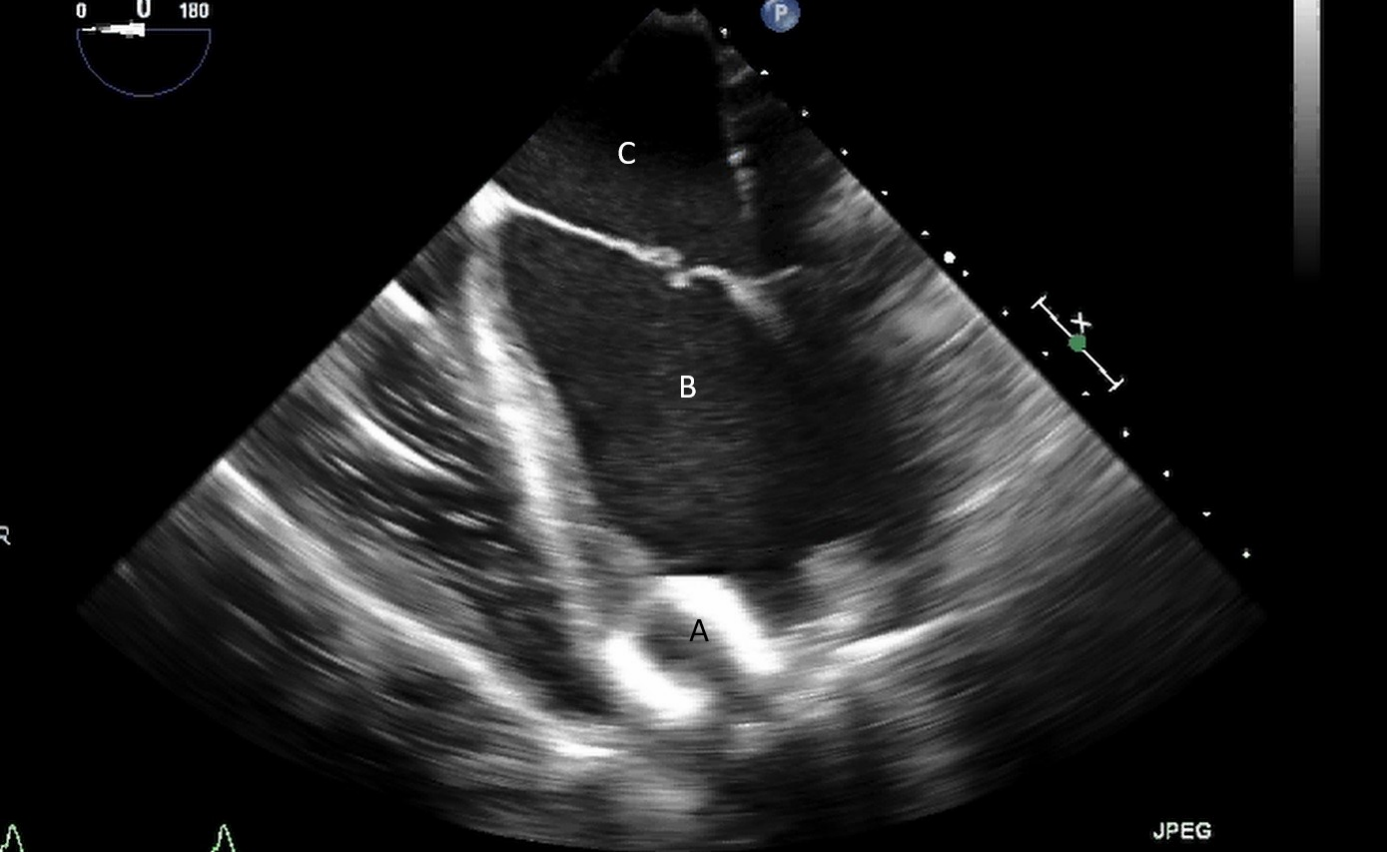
Transesophageal echocardiogram four chamber image with the LVAD
